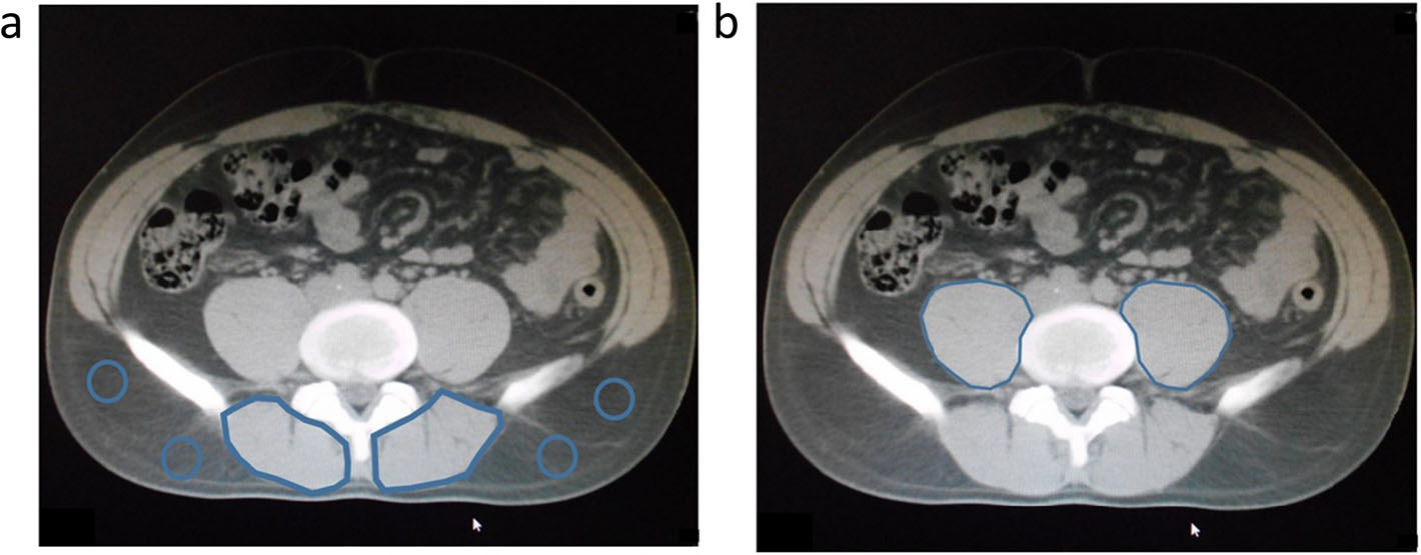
a Cross-sectional computed tomography imaging of subcutaneous fat area and subfascial muscular tissue in multifidus muscle. b Bilateral psoas muscles at level of umbilicus
